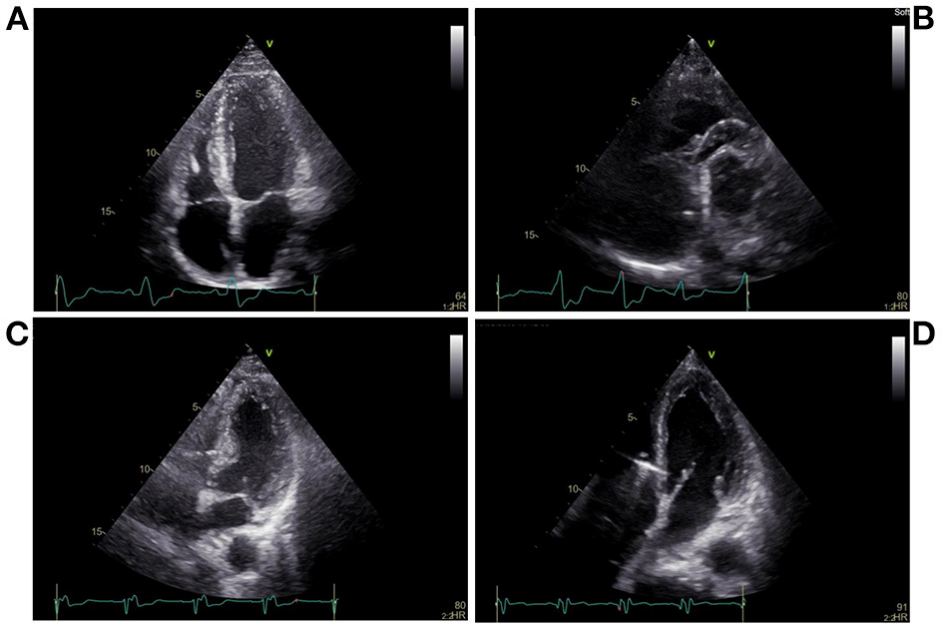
Echocardiography during pacing at different sites. (A) Right ventricular apical pacing, (B) Right ventricular outflow tract pacing, (C) His bundle pacing, (D) Left bundle branch pacing.
